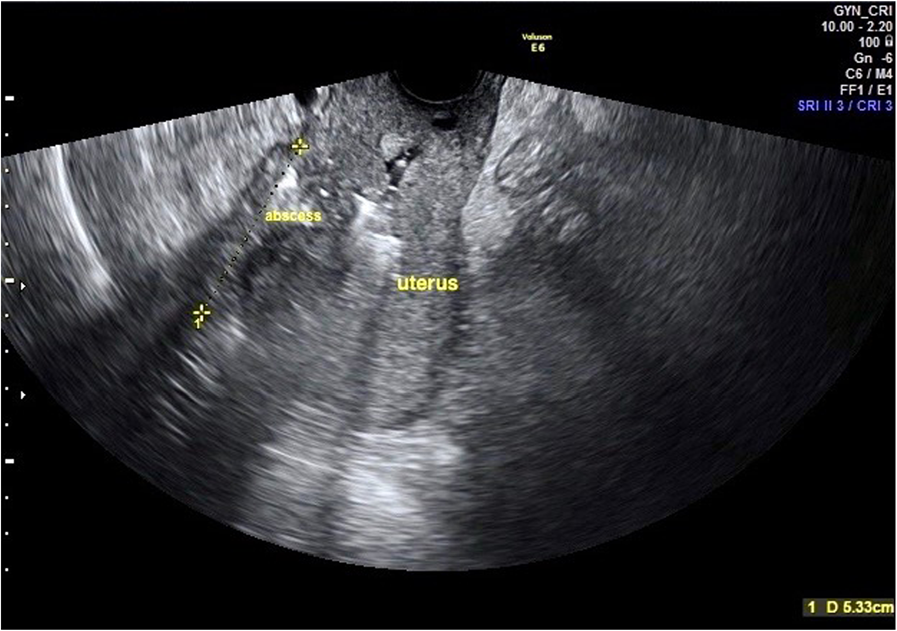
Pelvic Abscess at the ultrasound imaging. Abscess neighboring to the lower anterior wall of the uterus with dimensions of 53 x 47 mm
